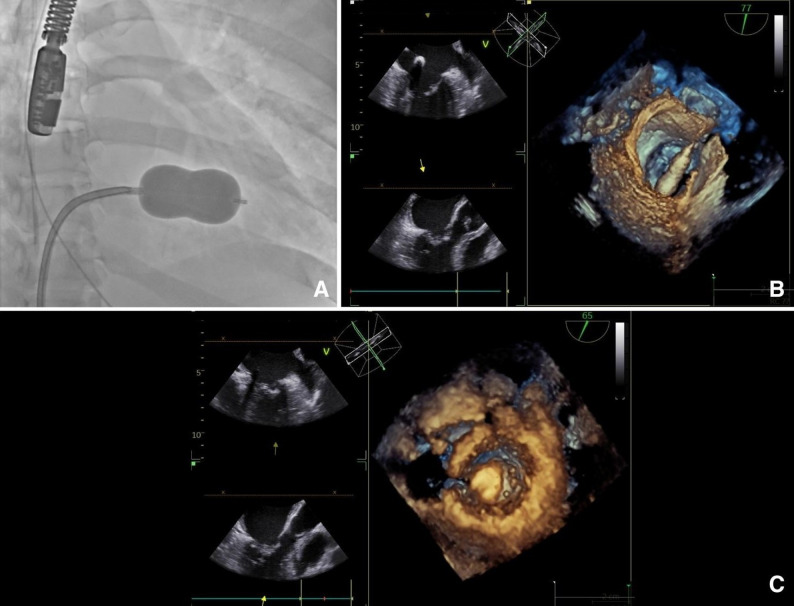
Percutaneous mitral commissurotomy. A Fluoroscopy showing inflation of a properly positioned valvuloplasty balloon. B Transesophageal echocardiogram (TEE), 77°. Advancement of balloon valvuloplasty catheter. C TEE, 65°. The balloon is inflated, opening the mitral leaflets, separation post balloon inflation is shown here
